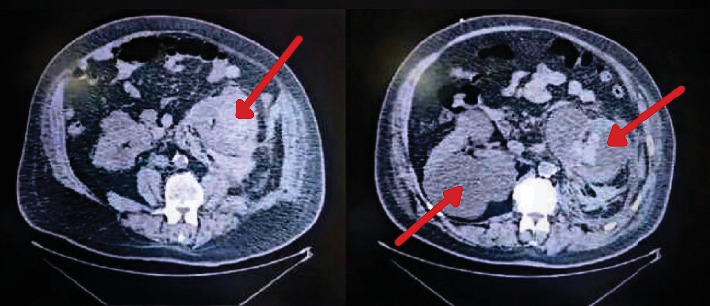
Contrast-enhanced CT scan demonstrating extensive perirenal hematoma associated with Wunderlich syndrome.
